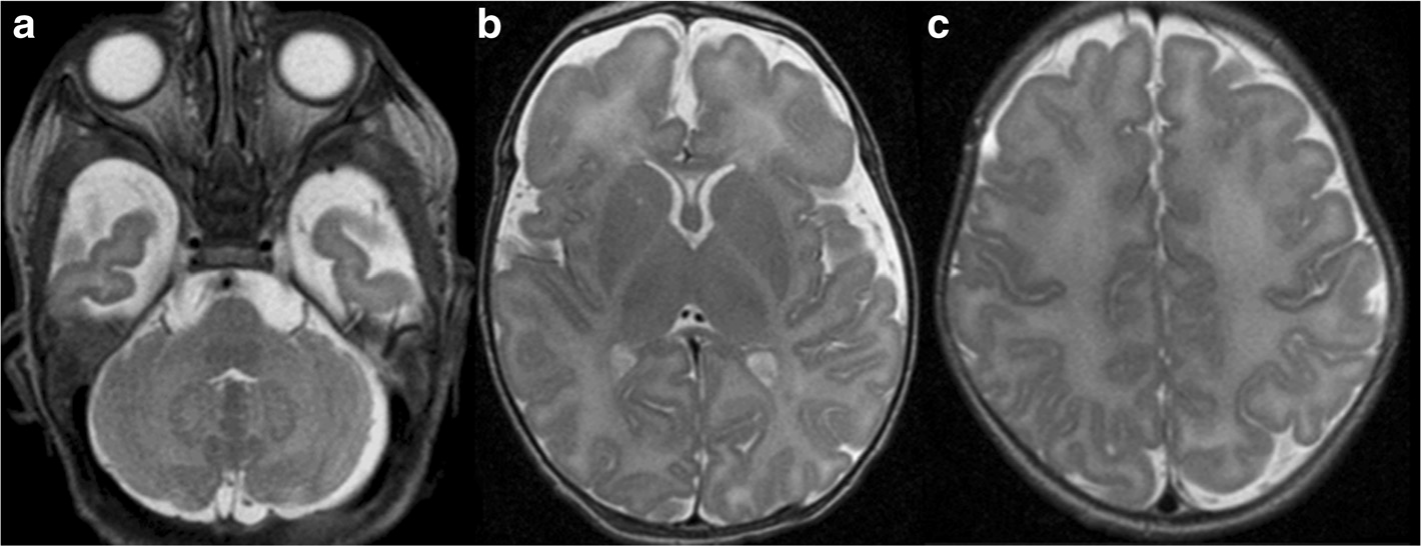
a-c, Axial T2-weighted MR images of a 5-day-old male term neonate with genetically confirmed Pelizaeus-Merzbacher disease presenting with marked muscular hypotonia, rotatory nystagmus, and inspiratory stridor show a homogeneous increased hyperintense signal of the supra- and infratentorial white matter including the corticospinal tracts within the posterior limb of the internal capsule bilaterally compatible with hypomyelination
